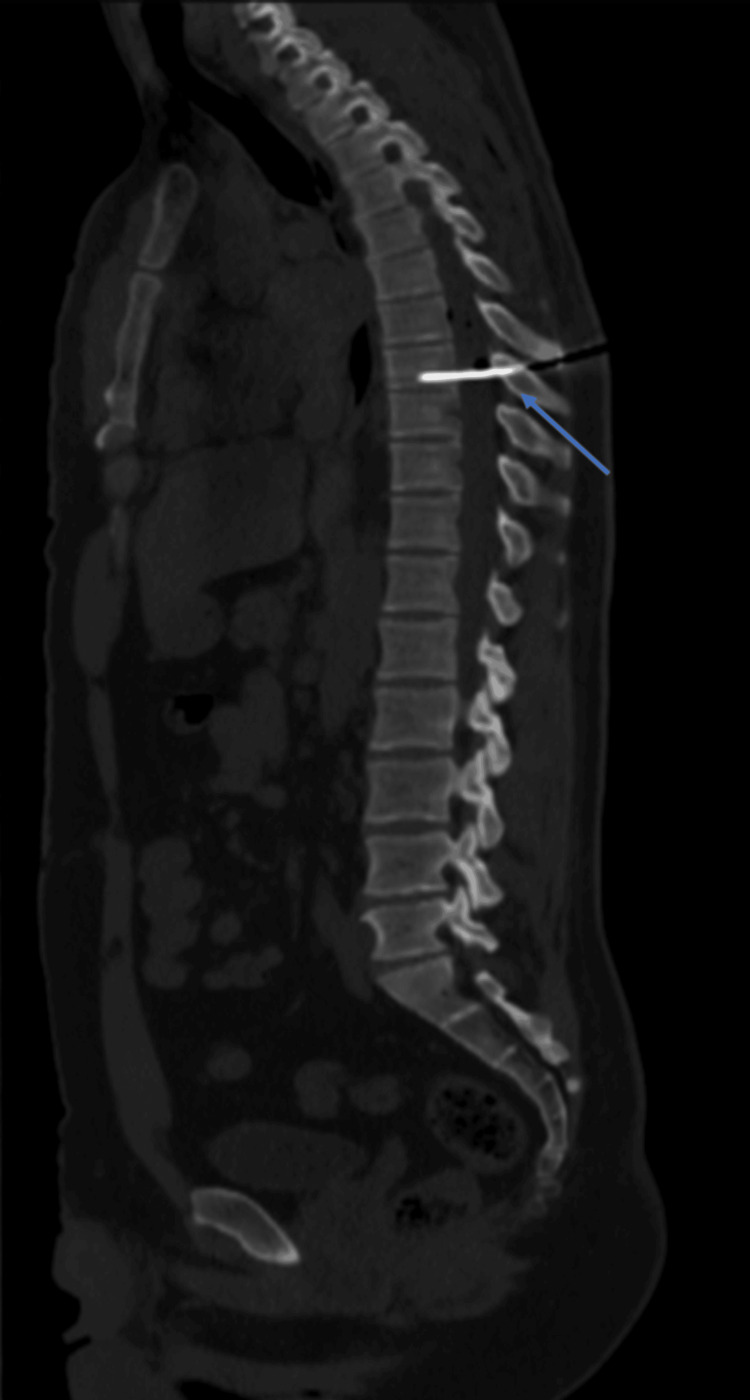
A computed tomography scan depicting the penetrating object crossing the T8 vertebral body (blue arrow).
